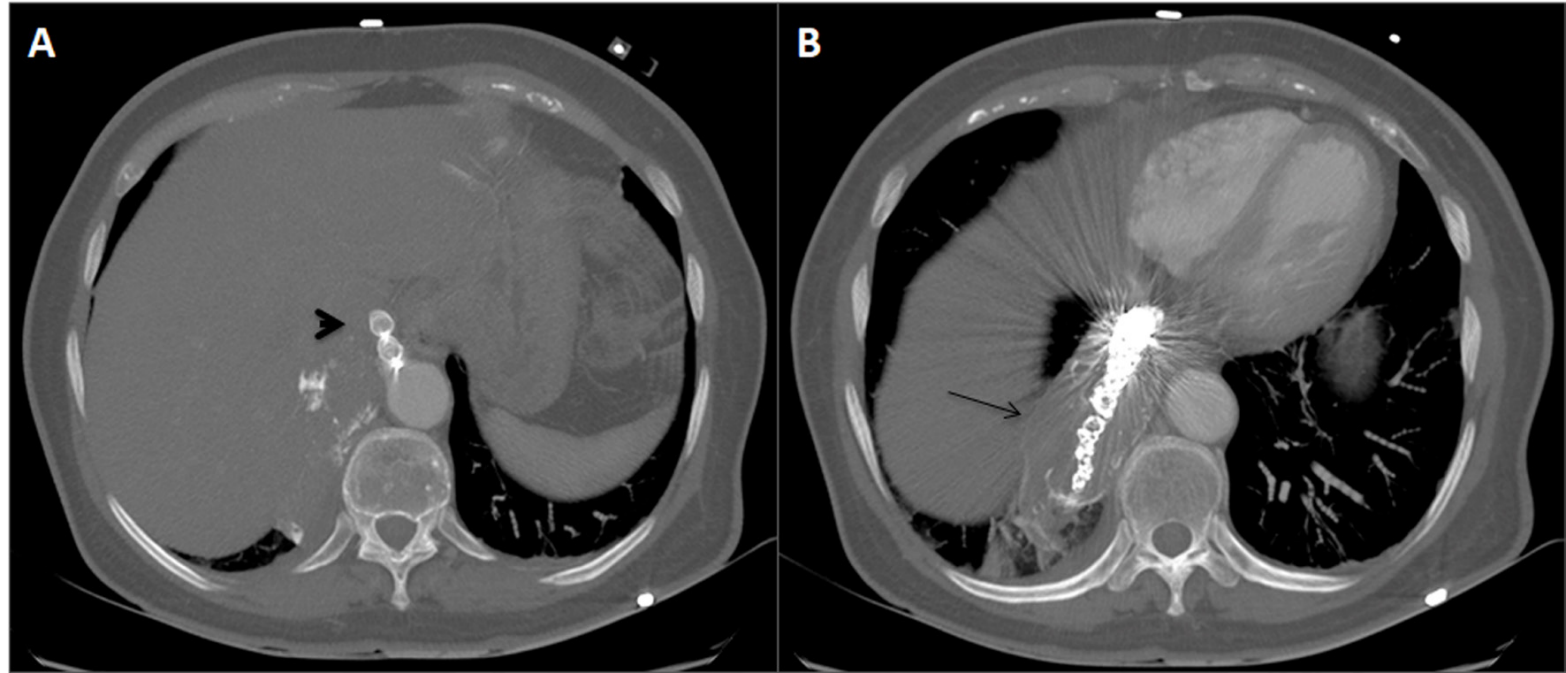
CTA performed before hospital discharge shows (A) the Amplatzer plug (arrowhead) and (B) the coils (black arrow), with absence of contrast and thrombus formation in the aneurysmal sac (black arrow in 4B).
